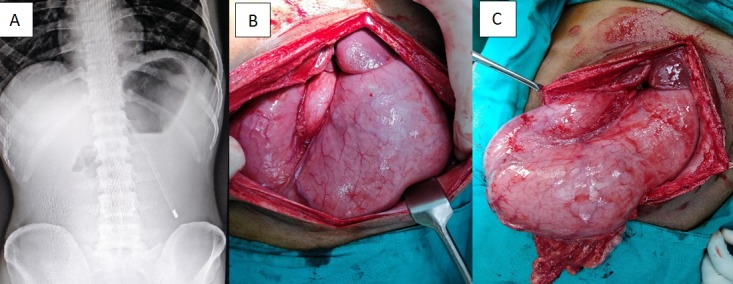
(A) X-Ray of the abdomen showing ground glass appearance with a single air-fluid level without any evidence of gas under both domes of diaphragm; (B) massively distended stomach covering almost all the quadrants of the abdomen; (C) massively distended stomach with no evidence of any peritoneal fluid collection
